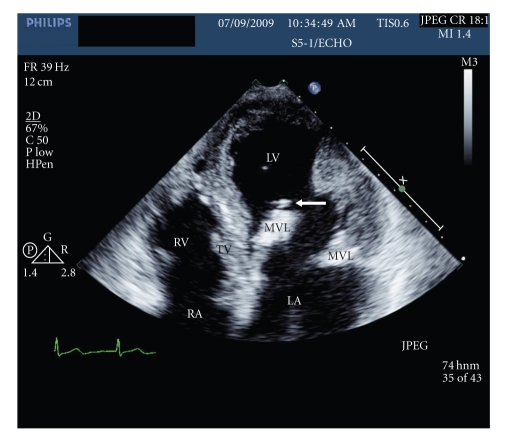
Four chamber view echocardiogram showing large vegetation on the MVL marked by arrow. LV-left ventricle, LA-left atrium, RV-right ventricle, RA-right atrium, TV-tricuspid valve, MVL-mitral valve leaflet.
